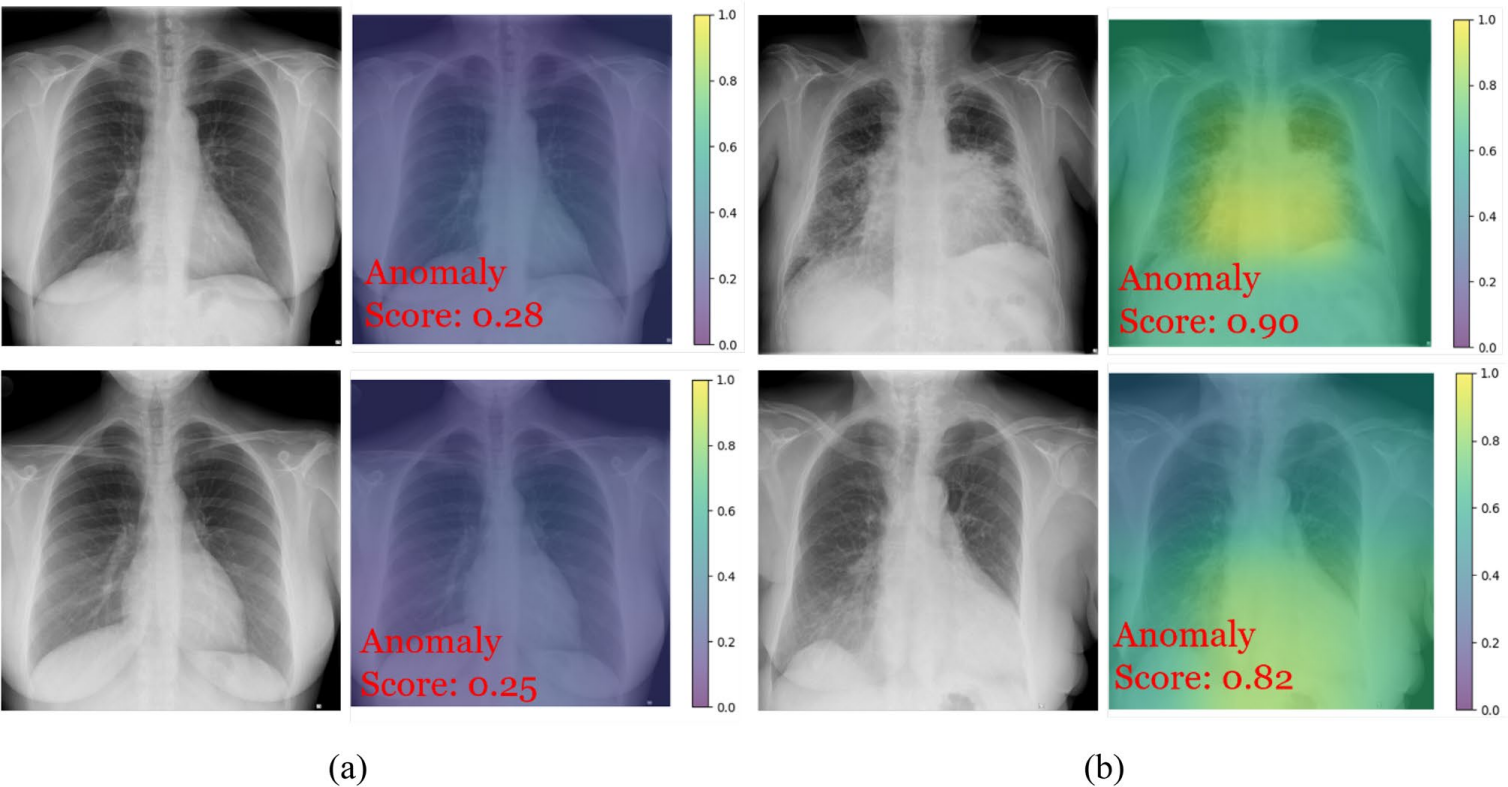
Radiographs of selected correctly classified examples—the anomaly scores were generated by the proposed method that showed the best performance in the experiments: (a) Truth label: normal, predicted label: normal; (b) truth label: abnormal, predicted label: abnormal.
